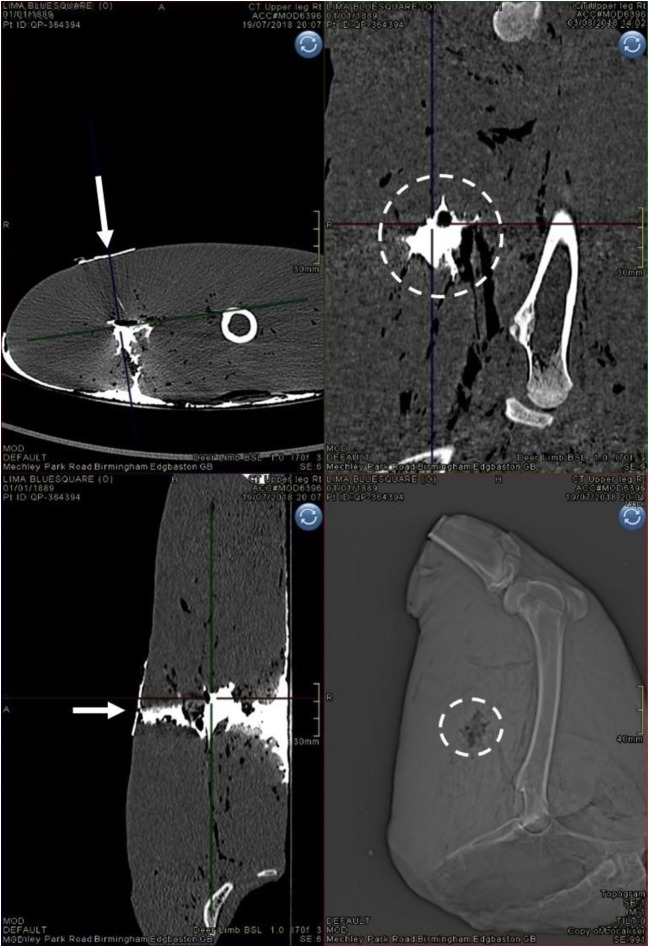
Arrows indicate projectile direction of travel, dotted circles indicate coronal section view of GSW track—clockwise from top left—contrast image, axial plane; contrast image, sagittal plane; CT scout view prior to contrast injection, sagittal plane; contrast image, coronal plane
